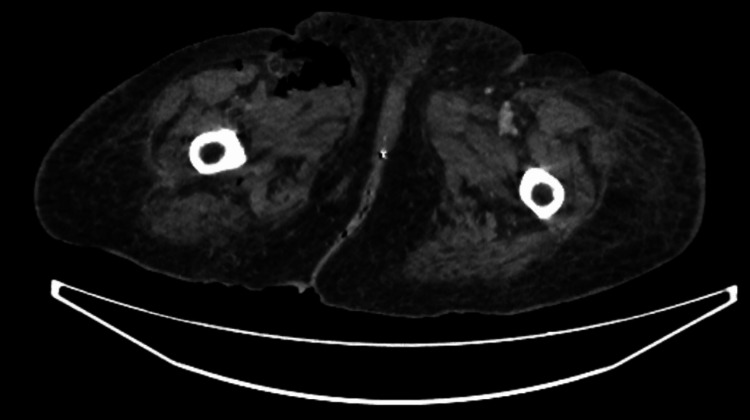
CT scan of both lower limbs showing gas in the right thigh.
